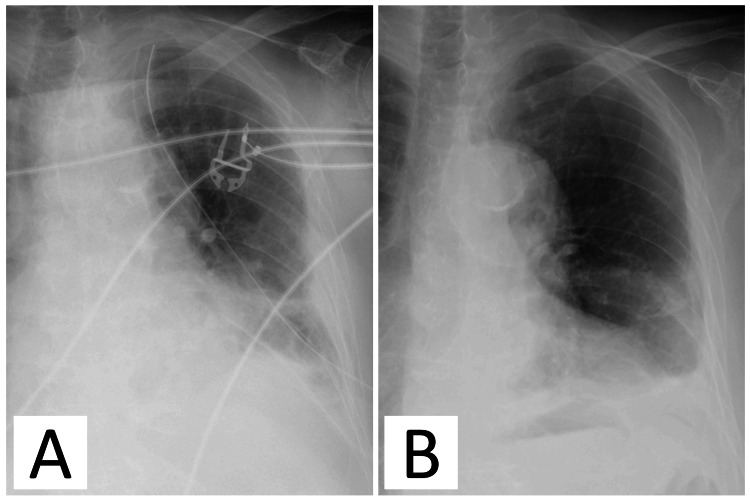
Postoperative imaging findings. (A) Chest x-ray on the day after surgery. (B). Final chest x-ray before discharge.
